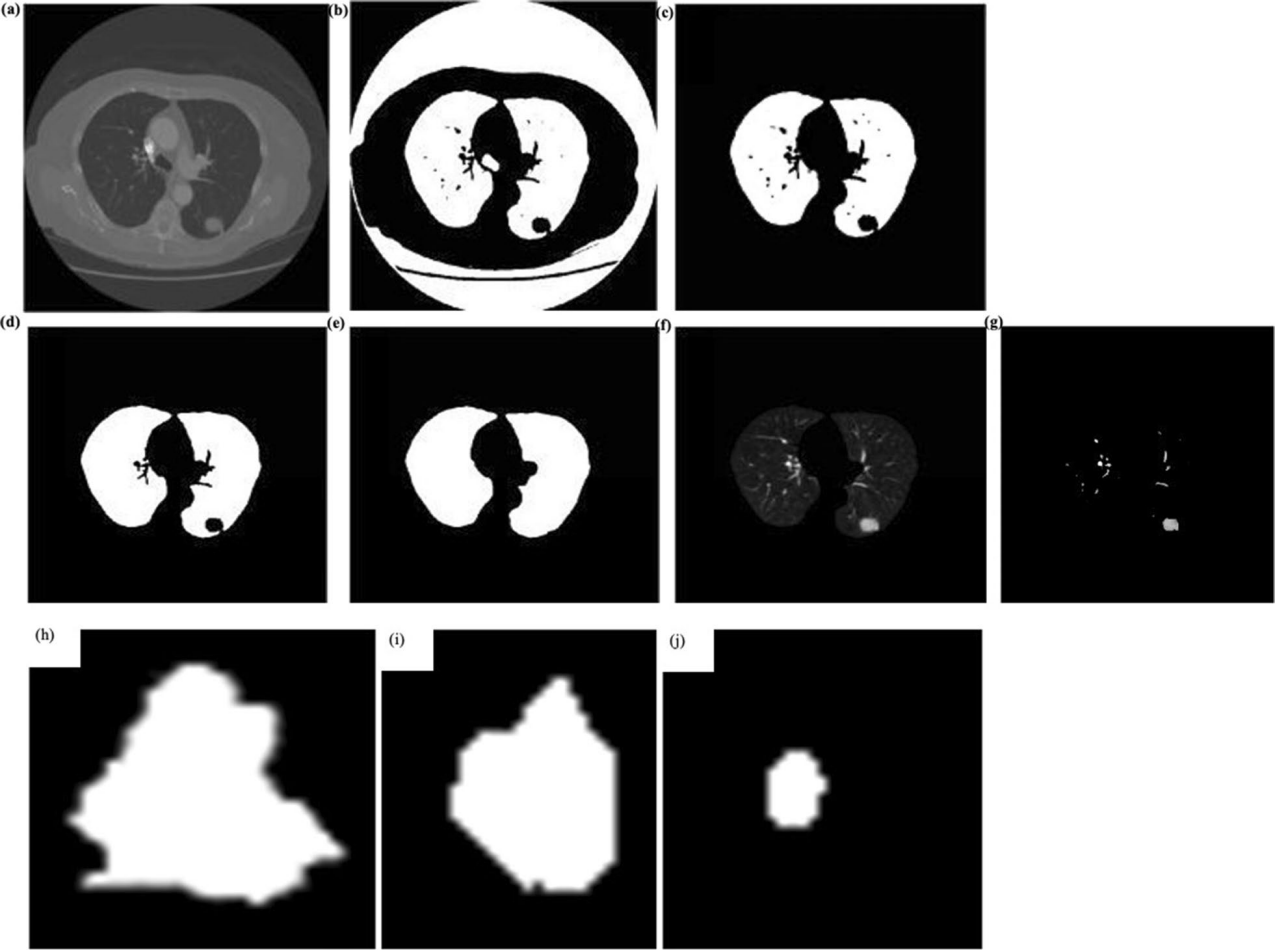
Classification and diagnosis. Results of preliminary segmentation, a CT gray image after Gauss filtering. b Initial segmentation of lung parenchyma. c Left/right lung extracted from initial segmentation of lung parenchyma Lung parenchyma segmentation based on the mathematical morphology, d holes filling, e boundary repairing, f final result of lung parenchyma, g possible pulmonary nodules located in segmentation region. h–j Sketch of some pulmonary nodules
